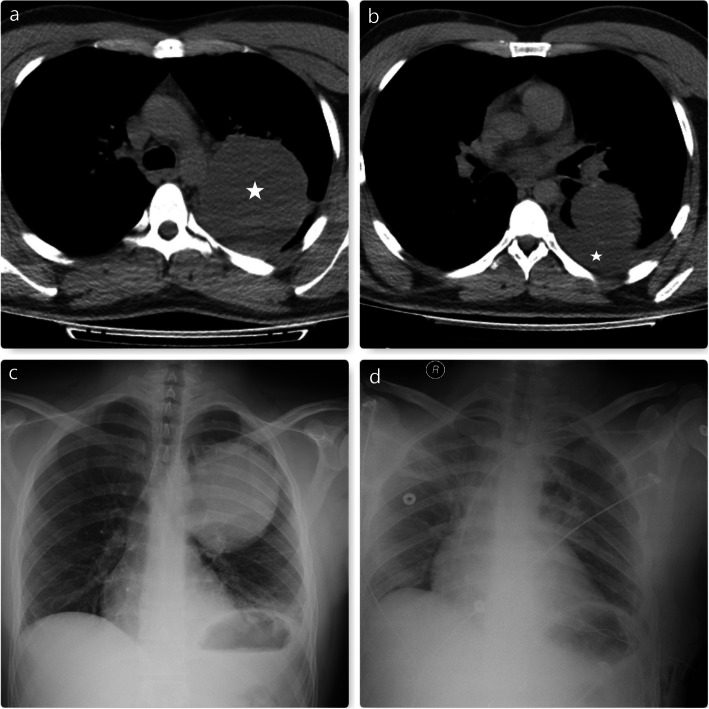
a. The left-sided giant bronchogenic cyst is seen in the thorax CT (Asterisk). b. This image shows pleural effusion accompanying bronchogenic cyst (Asterisk). Such effusions may occur due to atelectasis and disruption of lymphatic drainage, or they may be indicative of perforation in the cyst. In this case, perforation was detected intraoperatively. c and d indicate preoperative and postoperative chest radiograms
